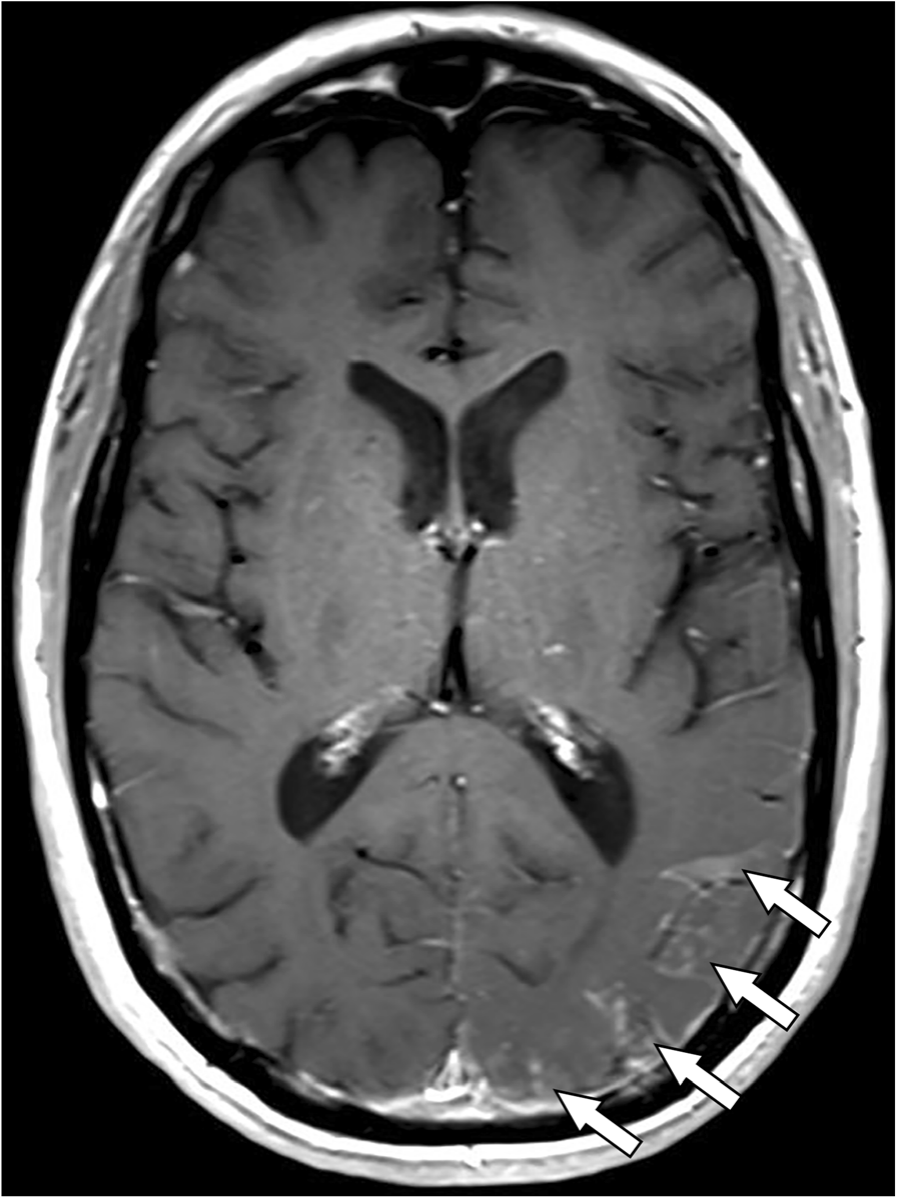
Brain MRI obtained at onset of neurological symptoms. T1-weighted contrast-enhanced axial image shows leptomeningeal enhancement involving the left occipital and parietal lobes (arrows)
